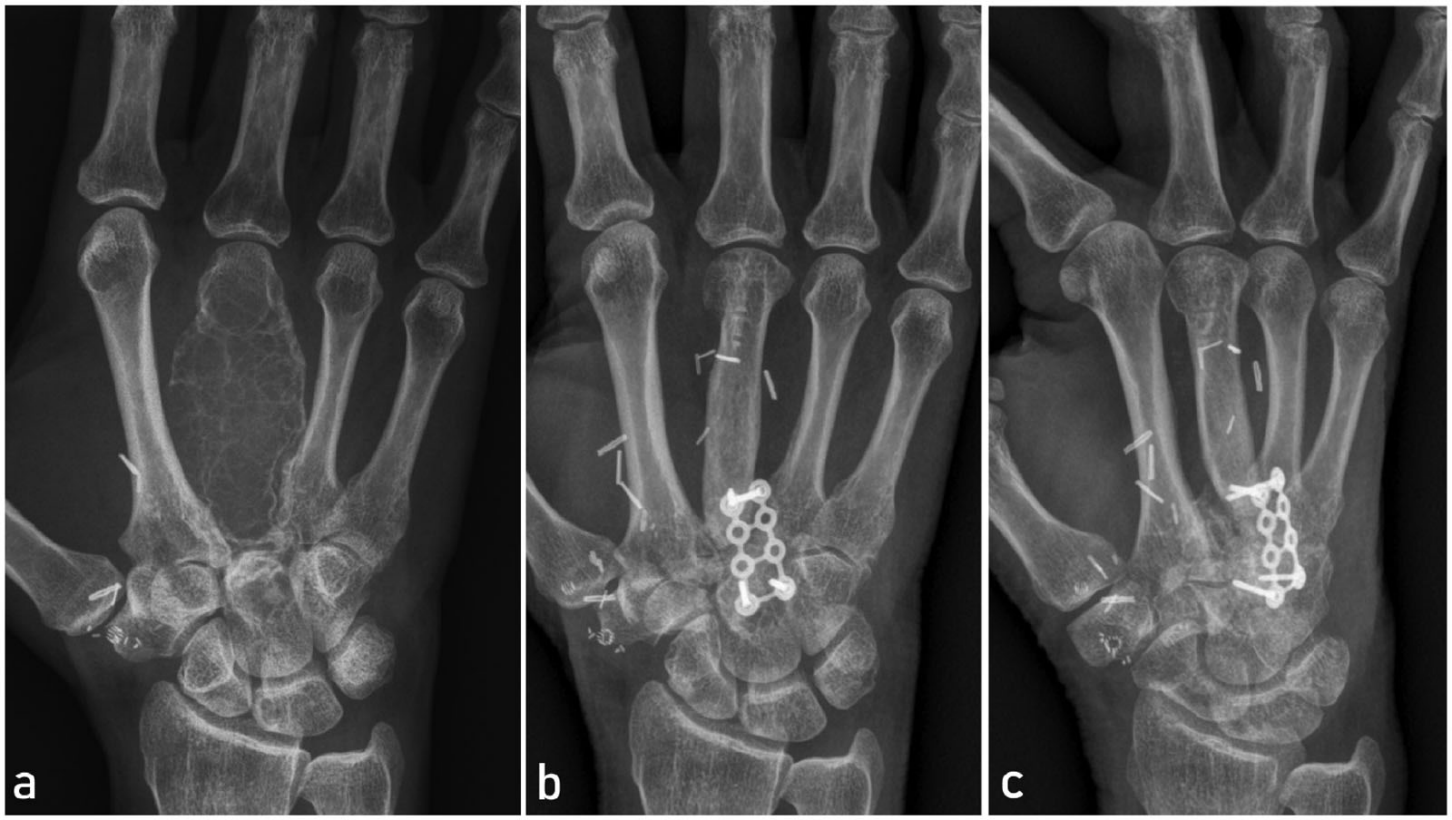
Preoperative x-ray showing the tumor encompassing the entire third right metacarpal including the metacarpal head (a). Follow-up x-rays one year after metacarpal reconstruction with MFC bone flap in dorsopalmar (b) and lateral view (c). Note the preservation of metacarpal length and distal bone remodeling at the metacarpal head.
